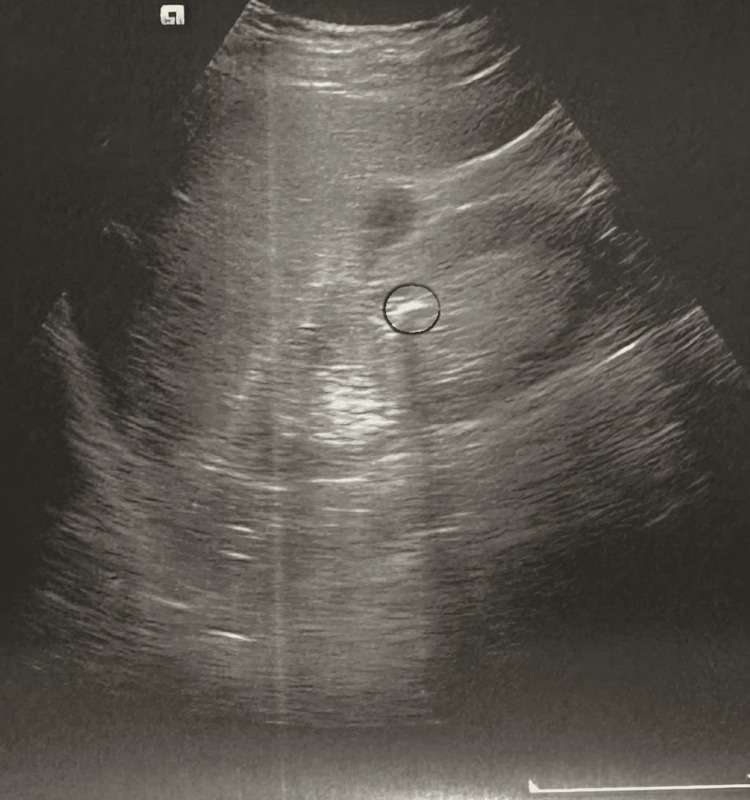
Echosonographic view of the kidneys of a patient who developed a nephrogenic bladder adenoma. The right kidney with an ultrasound image of a stone in the largest diameter of 6 mm shown with a circle
